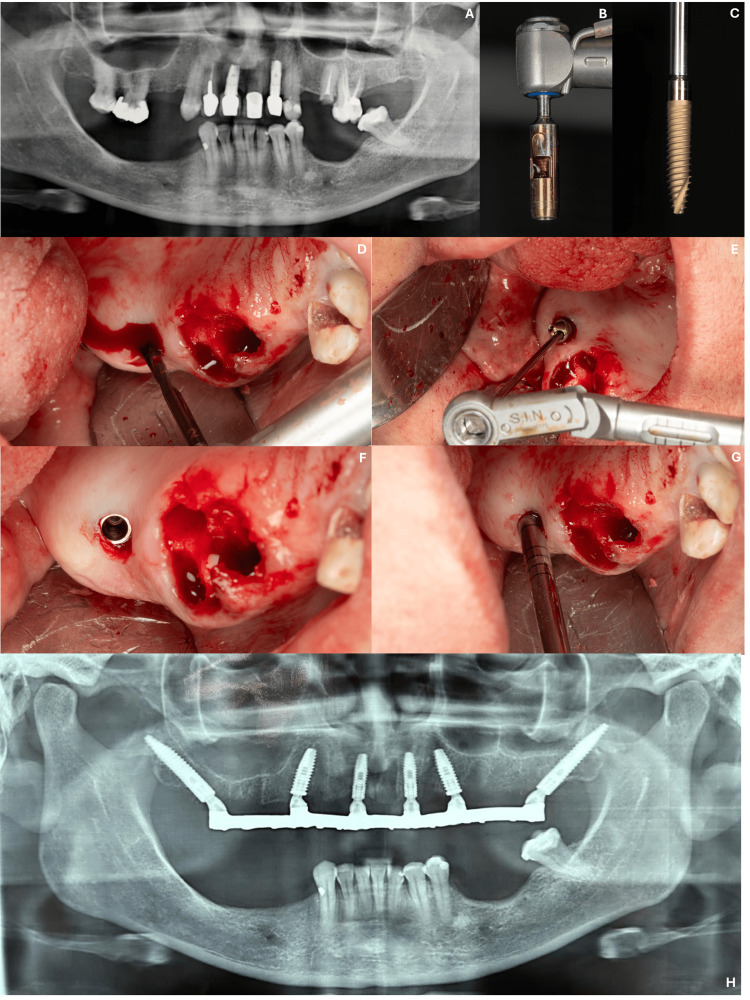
(A) Radiographic analysis showed that the patient presented a lot of teeth with an unfavorable prognosis; (B-G) four conventional implants and two pterygoid implants were placed to support a maxillary full-arch prosthesis; (H) radiographic condition of the prosthesis in function for 13 months without complications
